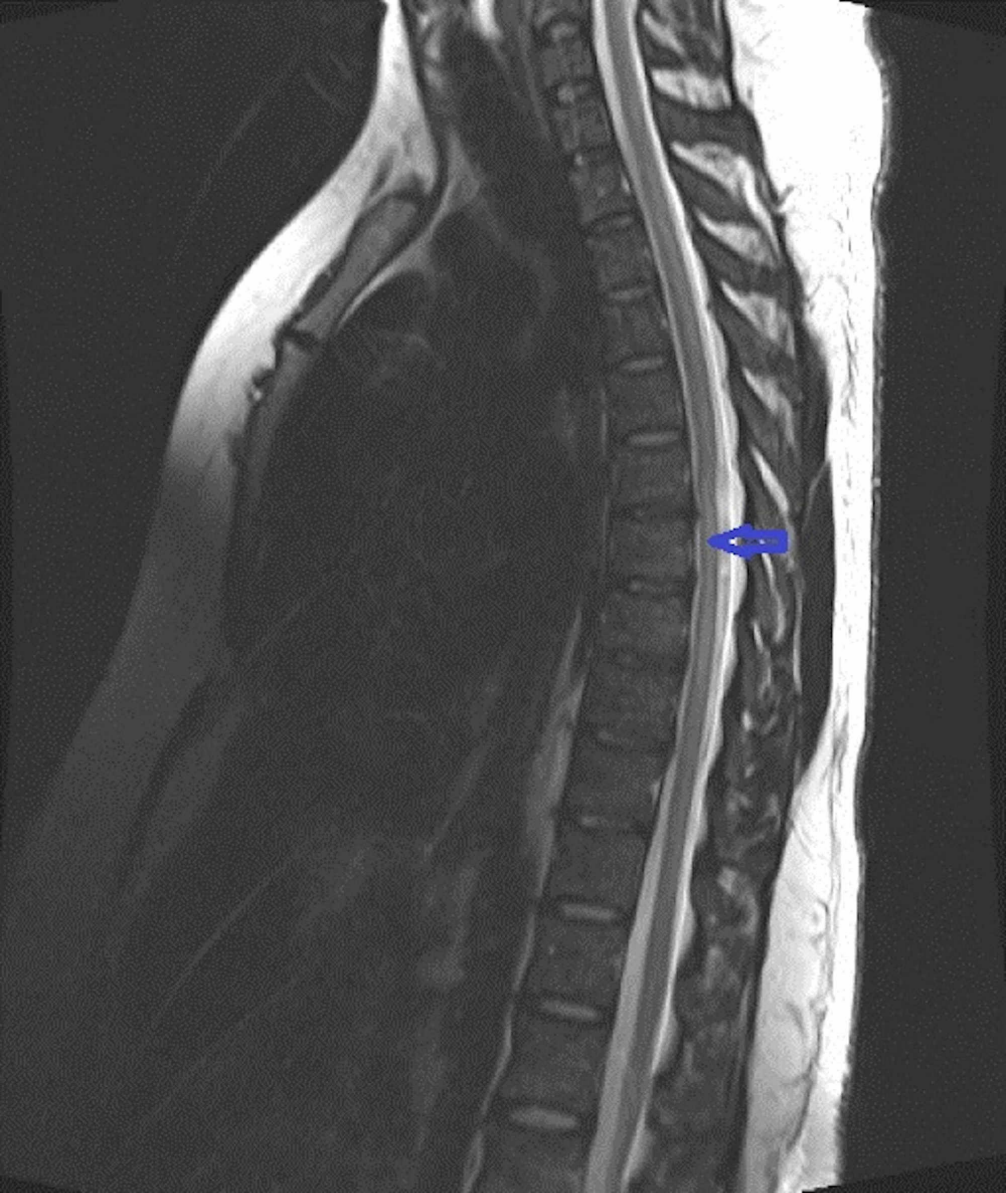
Magnetic Resonance Imaging (MRI) of thoracic spinal cord Sagittal T2- weighted MRI showed extra-medullary intradural hyper-intense signal in thoracic region (arrow).
